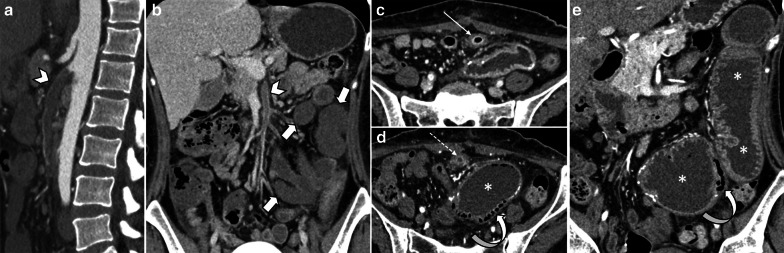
A 33 y/o female with anti-phospholipid antibody syndrome with an extensive SMA thrombosis (arrowheads) and proximal jejunal necrosis (thick arrows) (a, b). She presented with recurrent abdominal pain 45 days after bowel resection surgery. c Marked thickening of small bowel near anastomosis is depicted with mucosal enhancement (thin arrow) suggestive of early ischemic changes. Follow-up CT on day 57 (d, e) showed dilation of small bowel (asterisk) with pneumatosis (curved arrows) upstream to a late outcome fibrotic stricture (dotted arrow) that was confirmed at surgery
